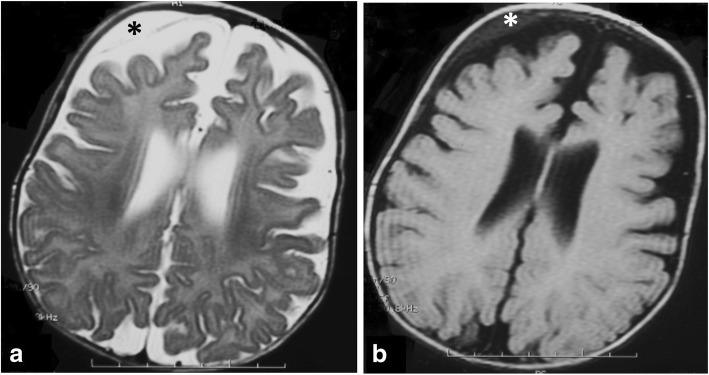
Axial T2-WI (a) and FLAIR (b) of a 5-month-old girl with glutaric aciduria and macrocephaly showing ventriculomegaly with subdural collection (asterisk)
